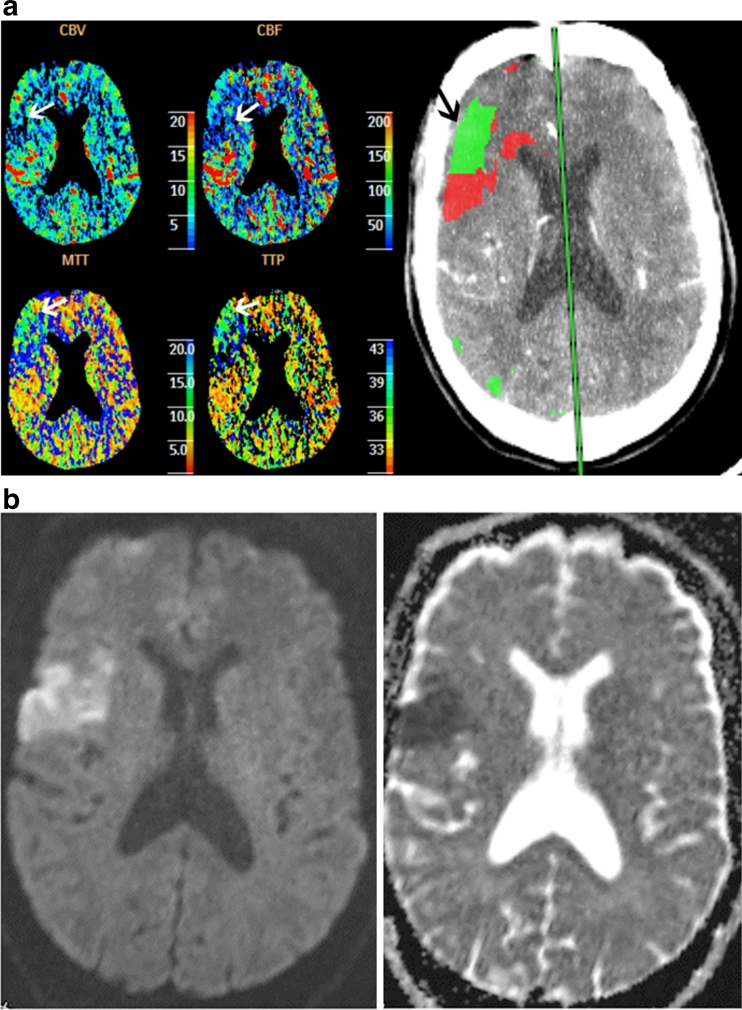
Sixty-five-year-old male presenting with slurred speech and facial droop. a Perfusion maps demonstrating a perfusion deficit (*open arrows*) with increasing areas from CBV to CBF to MTT/TTP. The corresponding computer-generated lesion map shows a mixed lesion (*closed arrow*). b MRI DWI and ADC map performed 4 h later demonstrate an area of decreased diffusion indicating acute infarct matching the area of decreased CBV on CT perfusion
